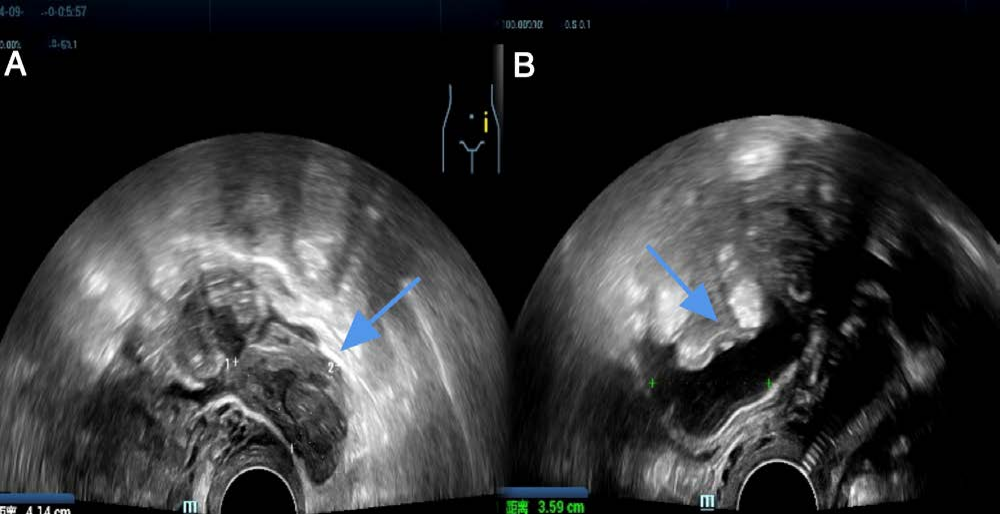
Preoperative transvaginal ultrasound findings. (A) Sagittal view showing a mixed echogenic mass (arrowheads) posterior to the uterine body, composed of heterogeneous solid and cystic components. (B) Transverse view demonstrating an anechoic fluid collection (arrowheads) surrounding the uterus, indicative of intraperitoneal bleeding.
